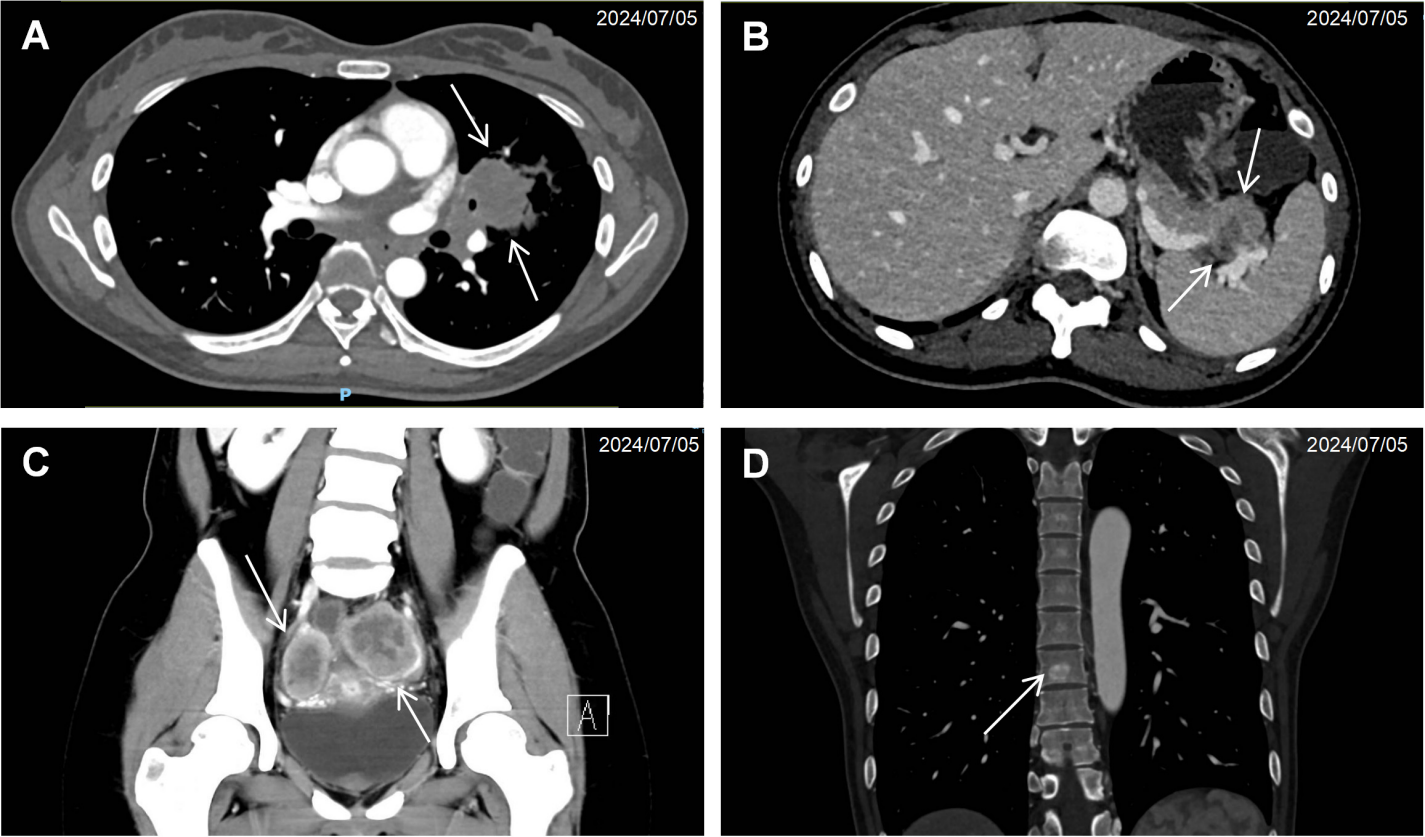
Cross-sectional imaging findings of neuroendocrine neoplasms. (A) Contrast-enhanced CT demonstrating a solid pulmonary mass (arrow) in the left upper lobe with associated bronchial truncation. (B) Hypodense pancreatic tail lesion (arrow) exhibiting ill-defined margins and poor demarcation from adjacent pancreatic parenchyma. (C) Bilateral adnexal complex cystic-solid lesions (arrow). (D) Anodular hyperdense lesion (arrow) within the T8 vertebral body.
